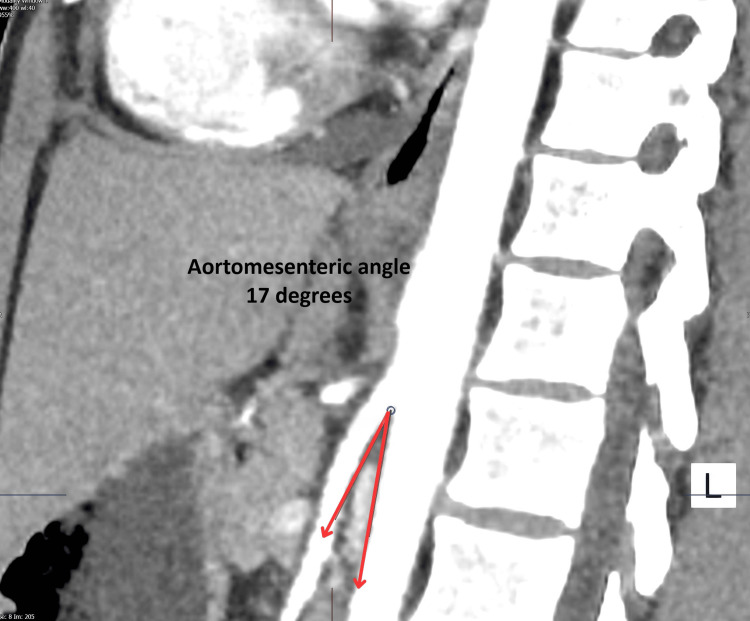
Sagittal contrast-enhanced CT image demonstrating a reduced aortomesenteric angle (17°) between the abdominal aorta and superior mesenteric artery, consistent with superior mesenteric artery syndrome. CT: computed tomography
